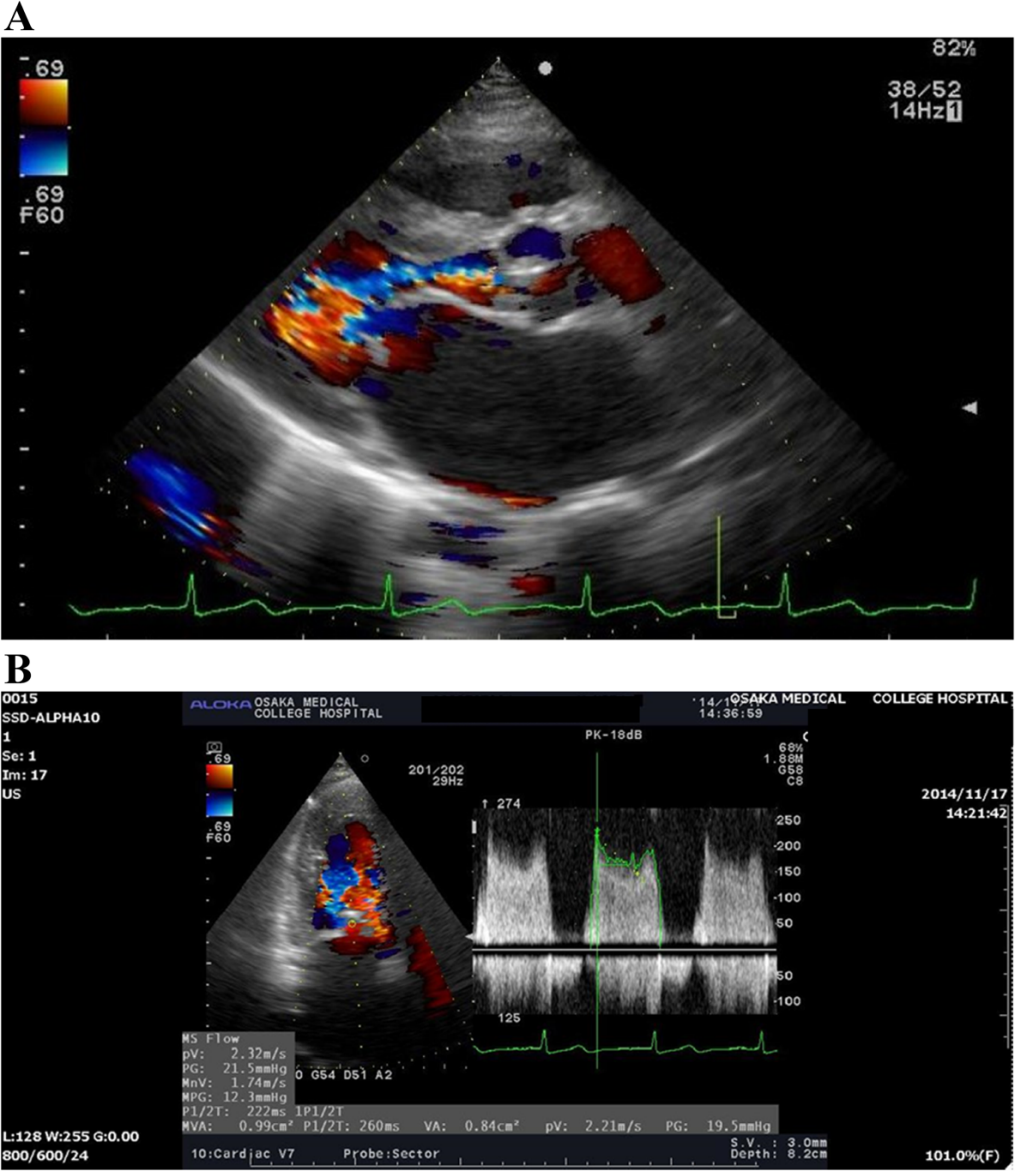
a Parasternal long-axis view with color Doppler assessment showing severe mitral valve stenosis. b Mitral valve area was 0.99 cm^2^ as measured by the pressure half-time method
